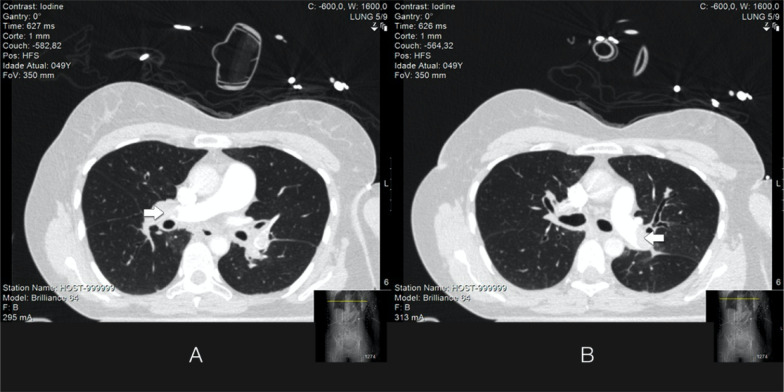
Computed tomography pulmonary angiogram showing major central pulmonary
